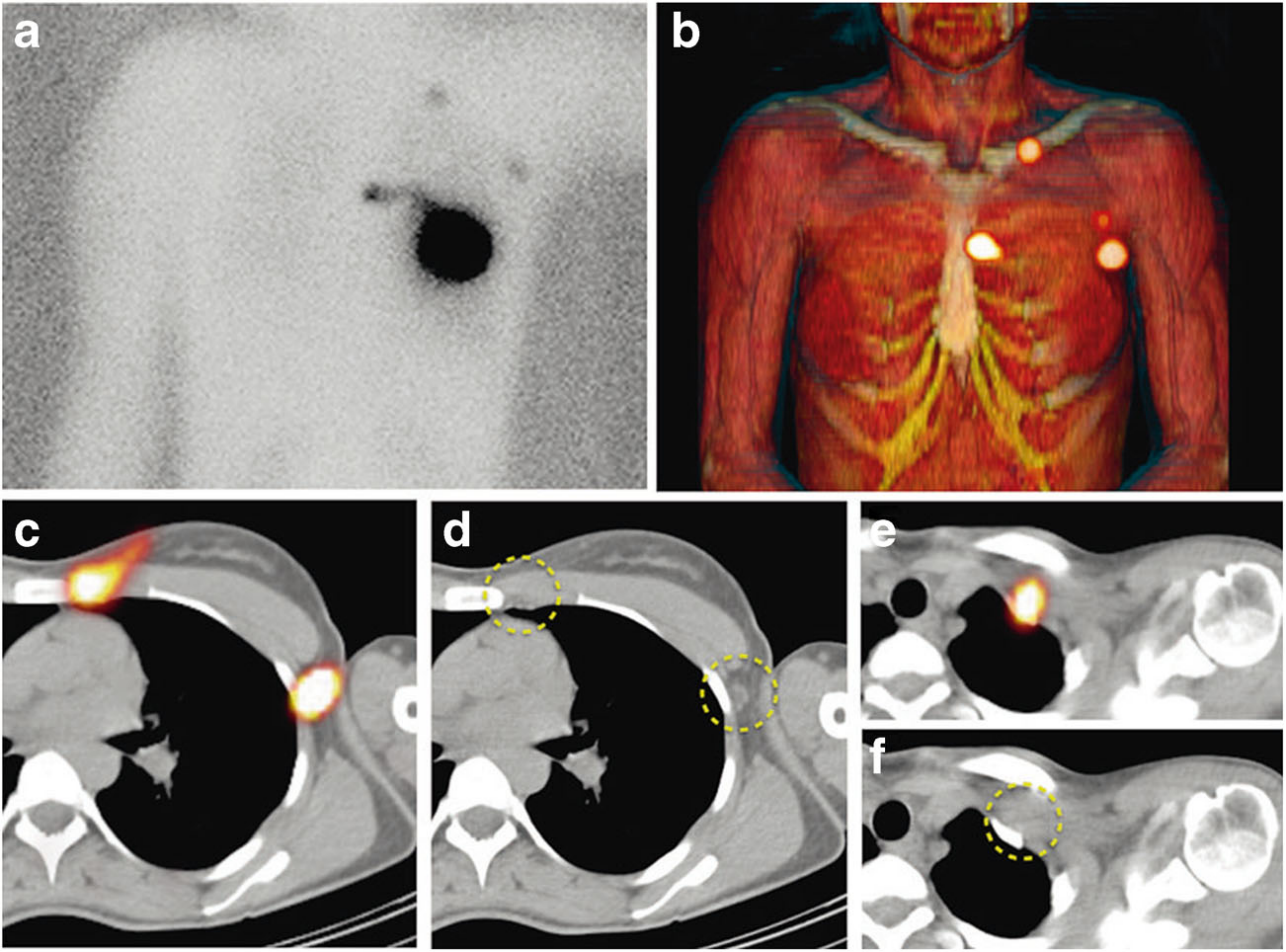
^99m^Tc-nanocolloid SPECT/CT in sentinel node lymphoscintigraphy. Preoperative sentinel lymph node (SLN) mapping in a 63-year-old woman with cancer of the left breast following intratumoral injection of ^99m^Tc-nanocolloidal albumin. Planar imaging with body contouring (a), with a ^57^Co flood source beneath the patient’s body, visualizes lymphatic drainage to SLNs in the left axilla, periclavicular area, and internal mammary chain. 3D surface volume rendering SPECT/CT (b) identifies the anatomical correlates of the SLNs localized on transaxial SPECT/CT and CT slices to the second intercostal space and level I of the left axilla, respectively (c, c), as well as behind the left clavicle (e, f), as indicated by dashed yellow circles. *[Reproduced with permission from: Giammarile F, Orsini F, Valdés Olmos RA, Vidal-Sicart S, Giuliano AE, Mariani G. Radioguided surgery for breast cancer. In: Strauss HW, Mariani G, Volterrani D, Larson SM, Eds. Nuclear Oncology – From Pathophysiology to Clinical Applications. New York: Springer; 2017:1363–1400]*
